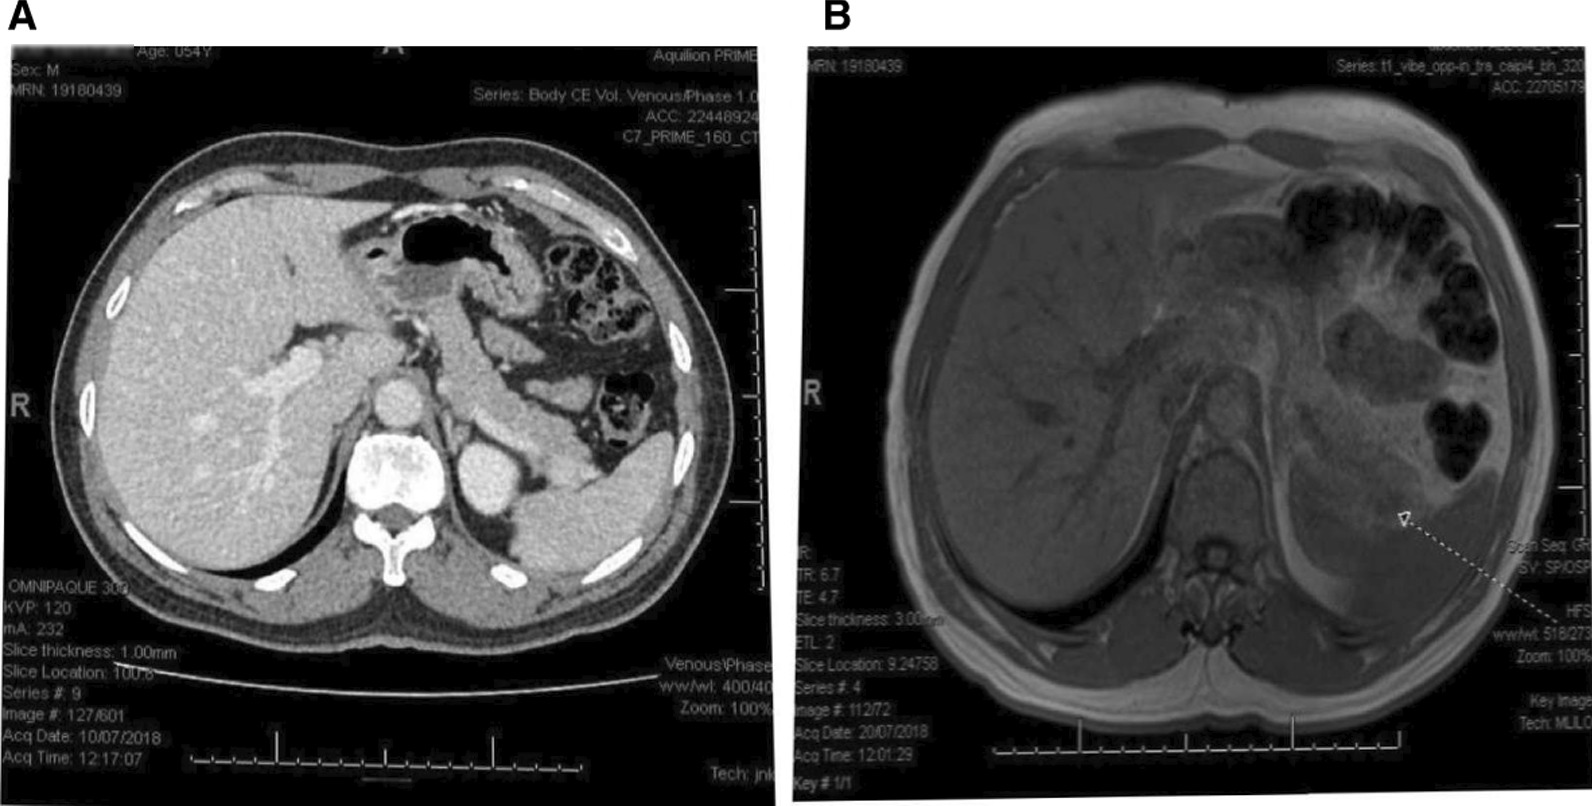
Contrast-enhanced computed tomography (CT) of the abdomen and magnetic resonance cholangiopancreatography (MRCP) of our patient. A CT of the abdomen showing a 12 × 10 mm mass lesion of the tail of the pancreas. B MRCP showing 10 × 12 mm mass in the pancreatic tail in keeping with an accessory spleen (arrow is indicating the lesion)
